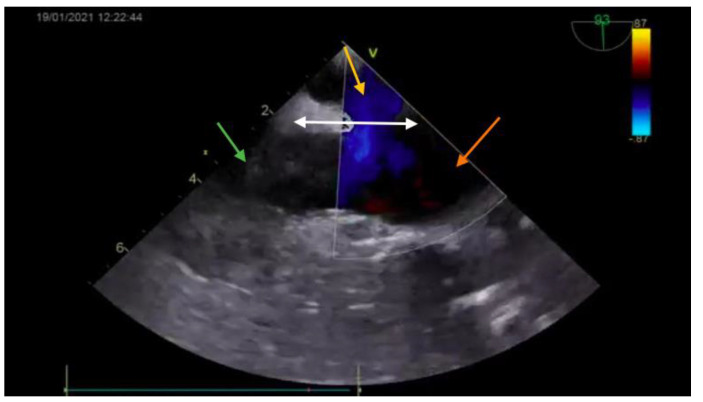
Preoperative echocardiography (bicaval view—Doppler; SVC—green arrow; right atrium—orange arrow; interatrial septum—white arrow) showing the ASD (yellow arrow).
